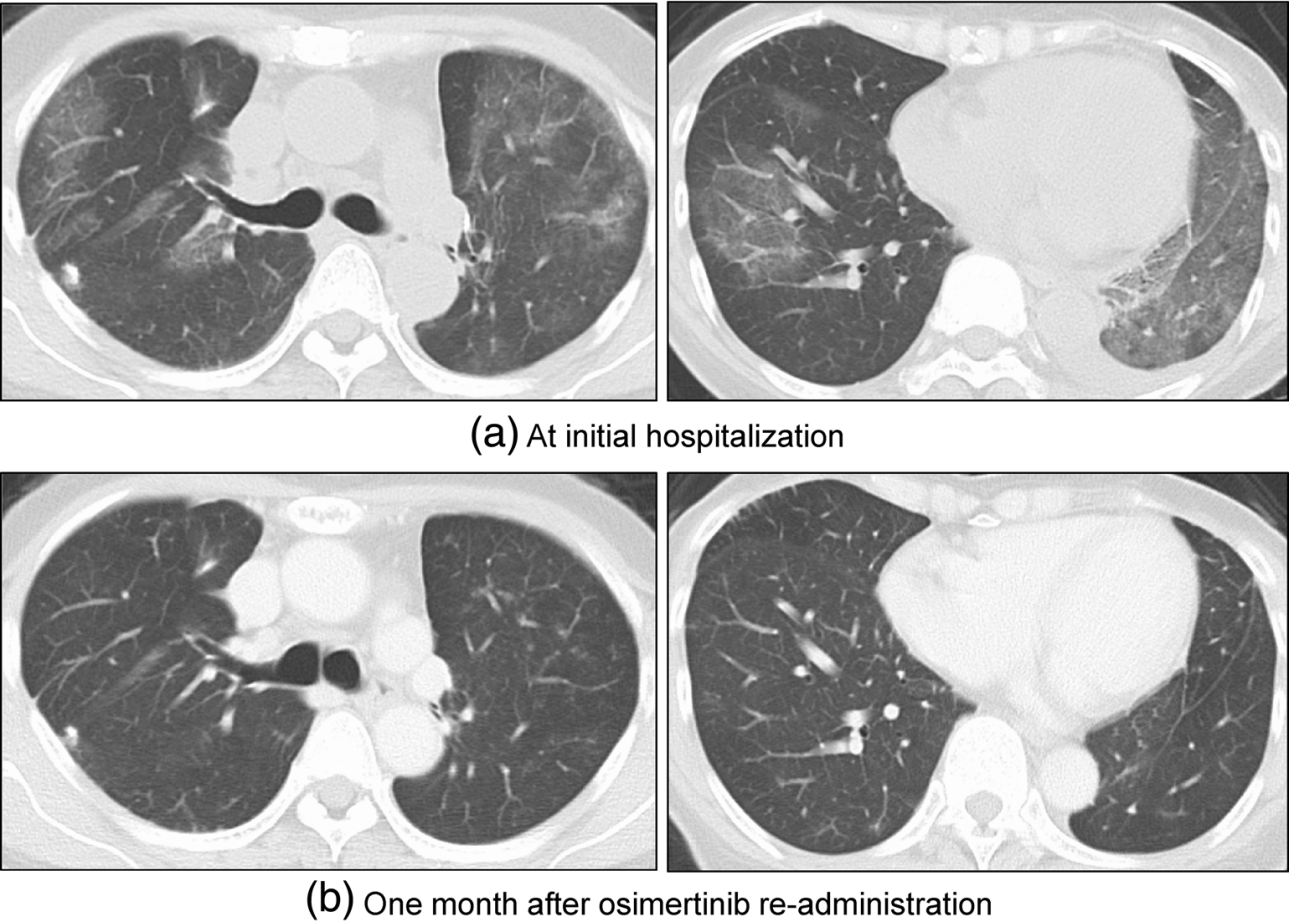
Chest computed tomography (CT) findings. (a) At the initial hospitalization for dyspnea, multiple ground‐glass opacities (GGOs) in both lung fields and new pleural effusion are observed. (b) One month after administration of osimertinib, the pleural effusion has resolved, and there is no evidence of interstitial lung disease recurrence.
